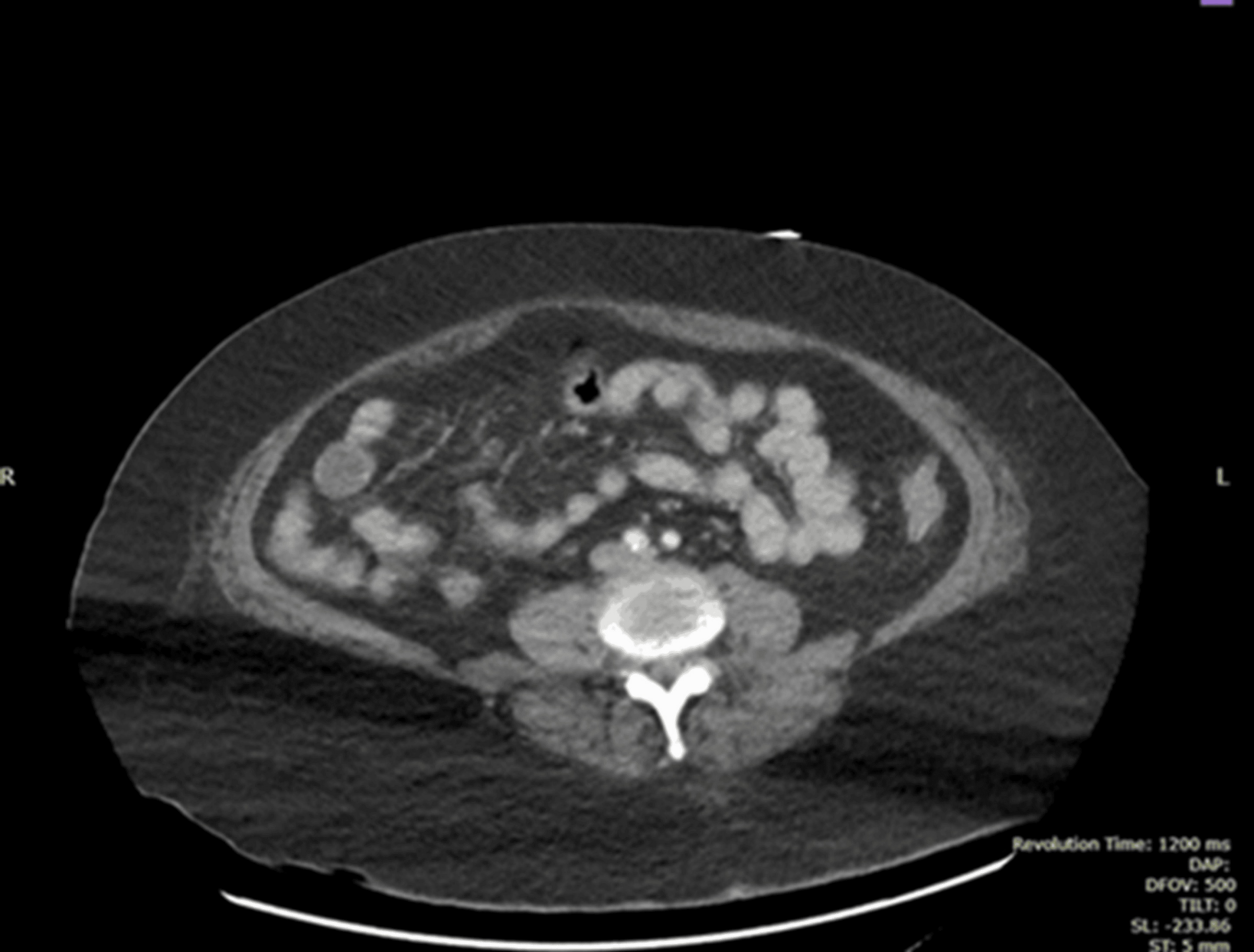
CT Abdomen with Contrast No intestinal ischemia
